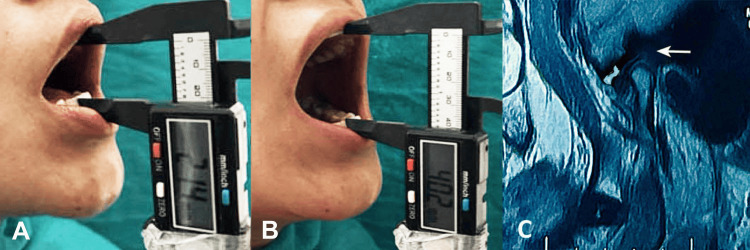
(A) Preoperative mouth opening (21.4 mm), (B) postoperative mouth opening (40.2 mm) at the three-month follow-up, and (C) MRI scan showing right temporomandibular joint effusion.
